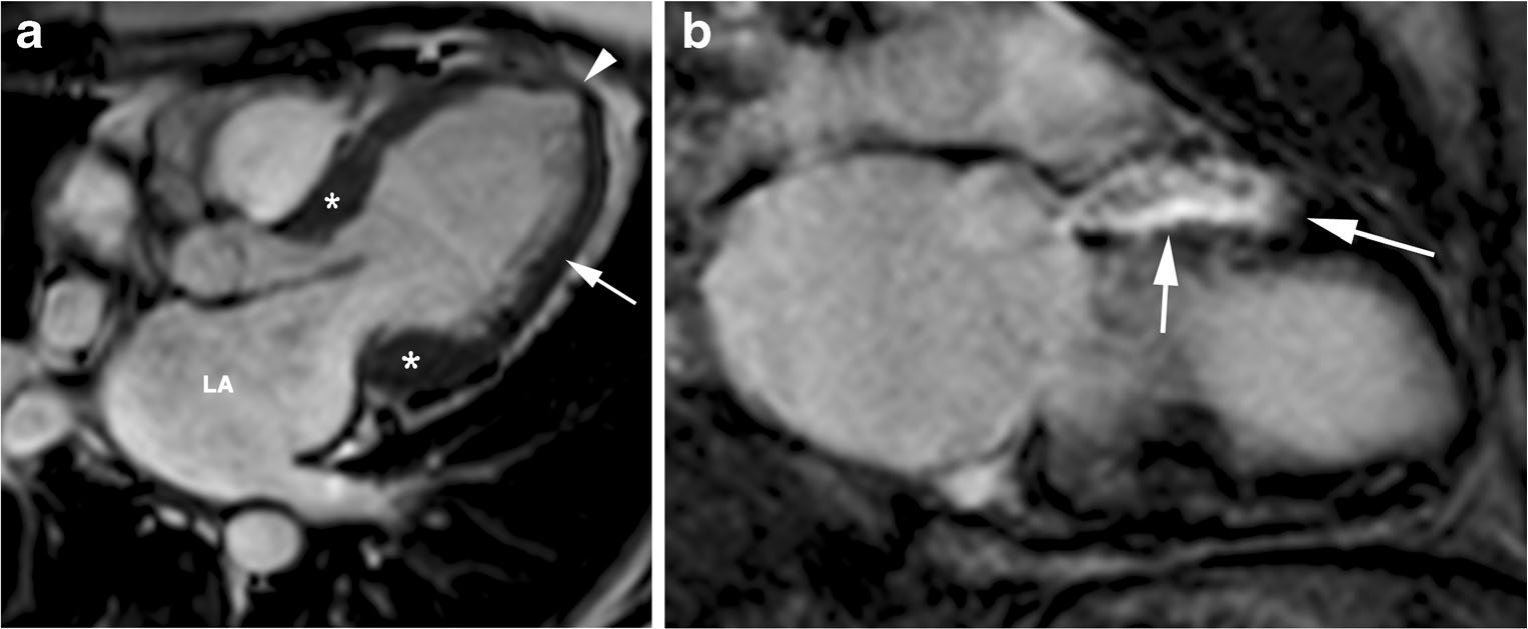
Adverse remodelling. 77-year-old man with long-standing asymmetric septal and lateral HCM who presented with increasing dyspnea on exertion and palpitations. a, End-diastolic horizontal long-axis SSFP cine MR image shows dilated and dysfunctional LV (ejection fraction, 45%), moderately thickened basal septum and lateral wall (15 mm) (asterisks), substantial thinning of the lateral LV wall (arrow), apical aneurysm (arrowhead) and dilated left atrium (LA). b, Short-axis inversion-recovery image shows transmural delayed enhancement in the anterior wall (arrows)
